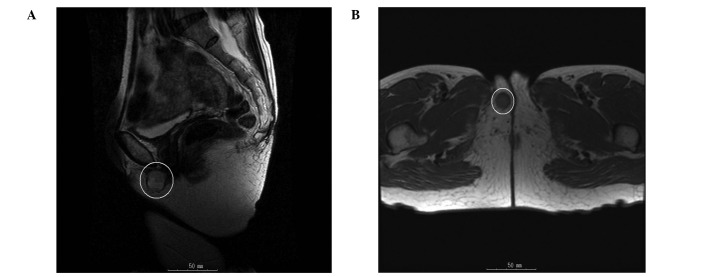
Pelvic magnetic resonance imaging revealed a 3-cm mass in the right side of the vulva. (A) Saggital T2-weighted images demonstrated high signal intensities and (B) Axial T1-weighted images demonstrated low signal intensities.
